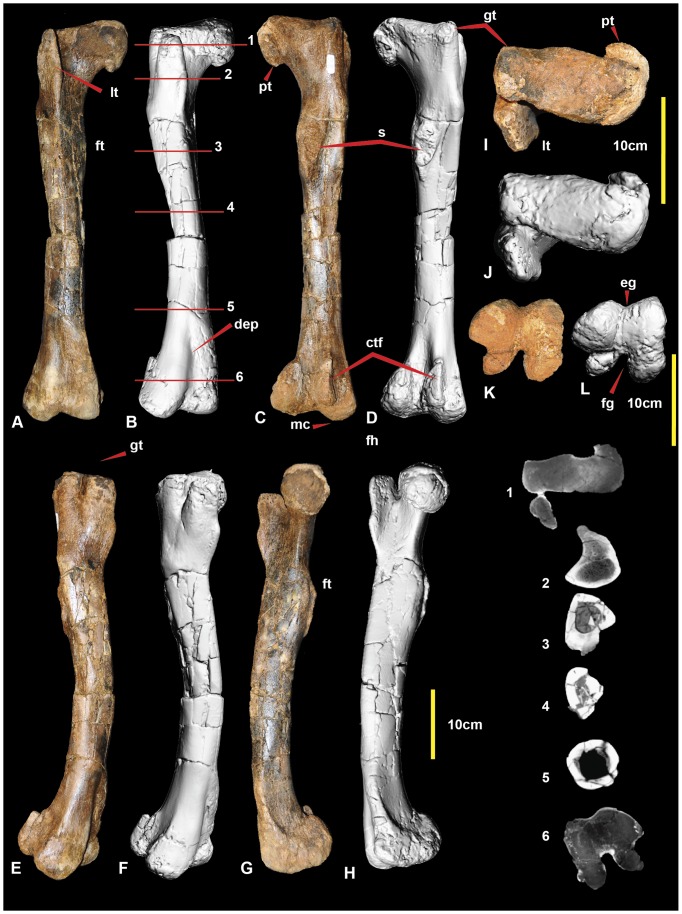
Right femur. Right femur in: cranial (A & B); caudal (C & D); lateral (E & F); medial (G & H); proximal (I & J); and distal (K & L) views. Abbreviations: ctf, crista tibiofibularis; dep, depression; eg, extensor groove; fg, flexor groove; ft, fourth trochanter; gt, greater trochanter; lt, lesser trochanter; mc, medial condyle; cf, caudal flange on caput; s, scar; 1-6 CT sections.
